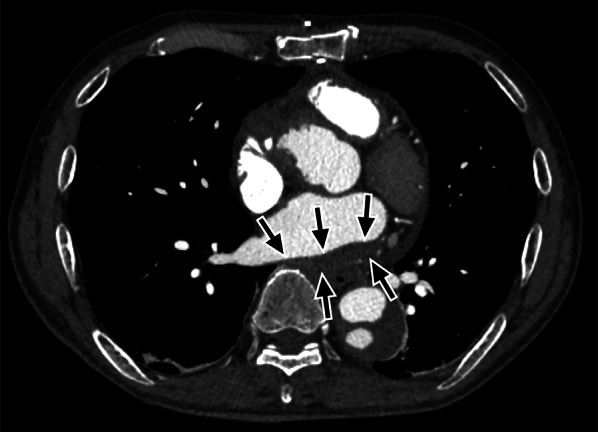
Follow-up imaging findings. Enhanced computed tomography on postoperative day 37 showing resolution of the left atrial dissection cavity (arrows)
